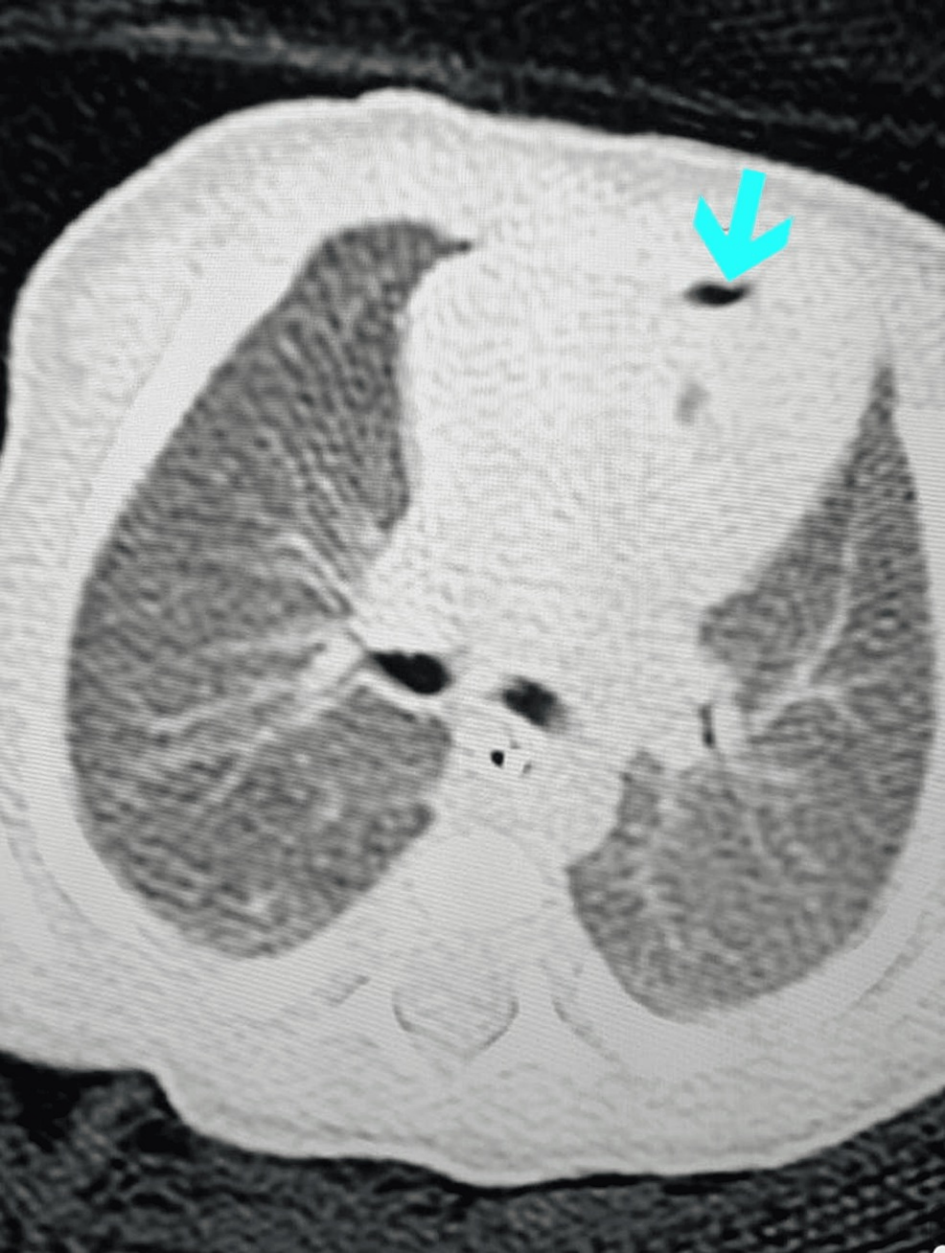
Chest (CT) scan demonstrated and revealed multiple tiny pockets of gases involving the anterior mediastinum which represents a pneumomediastinum. Grossly, there was no evidence of pericardial effusion and no pleural effusion.
